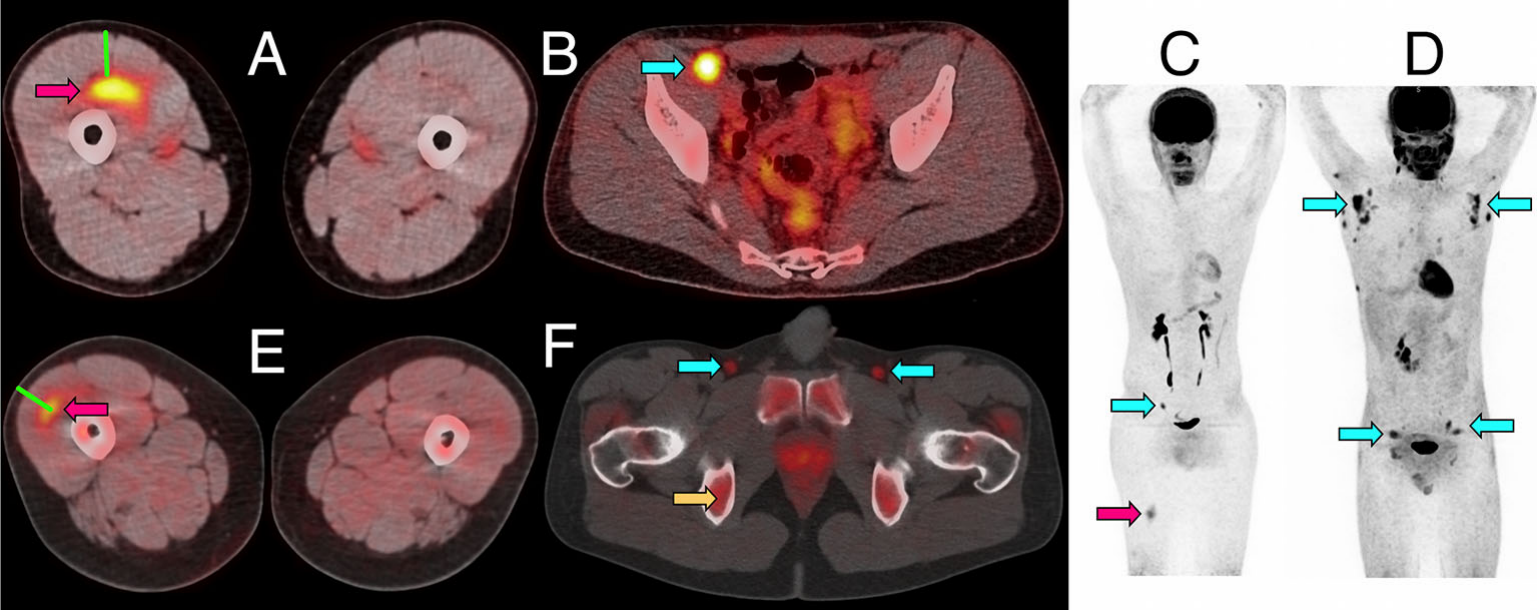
Representative PET/CT images of intramuscular injection site and draining lymph nodes. (A–C) Fused ^18^F-FDG PET/CT images (linear scale) from one participant 5 days after immunization with 4CMenB, demonstrating increased activity at right anterior thigh intramuscular injection site (A, red arrow) and right external iliac lymph node (B, cyan arrow); whole body PET scan anterior view (C) demonstrating level of injection site (red arrow) and activated lymph node (cyan arrow). (D) Whole body ^18^F-FDG PET scan anterior view demonstrating disseminated axillary and pelvic lymph node activity (cyan arrows) 1 day after immunization with ATIV. (E, F) Fused ^11^C-PBR28-PET/CT images (linear scale) from two participants immunized with 4CMenB demonstrating increased ^11^C-PBR28 binding in right thigh injection site (E: red arrow, day +3); and right and left deep inguinal lymph nodes (F: cyan arrows, day +7, right thigh injected). Increased bone marrow binding of ^11^C-PBR28 also visible (F: yellow arrow). Green bars (A, E) indicate length of immunization needle, actual track unknown. Diffuse uptake of ^18^F-FDG can be seen in bowel (B), and for both radionucleotides in urinary collecting system and bladder (B–D, F).
